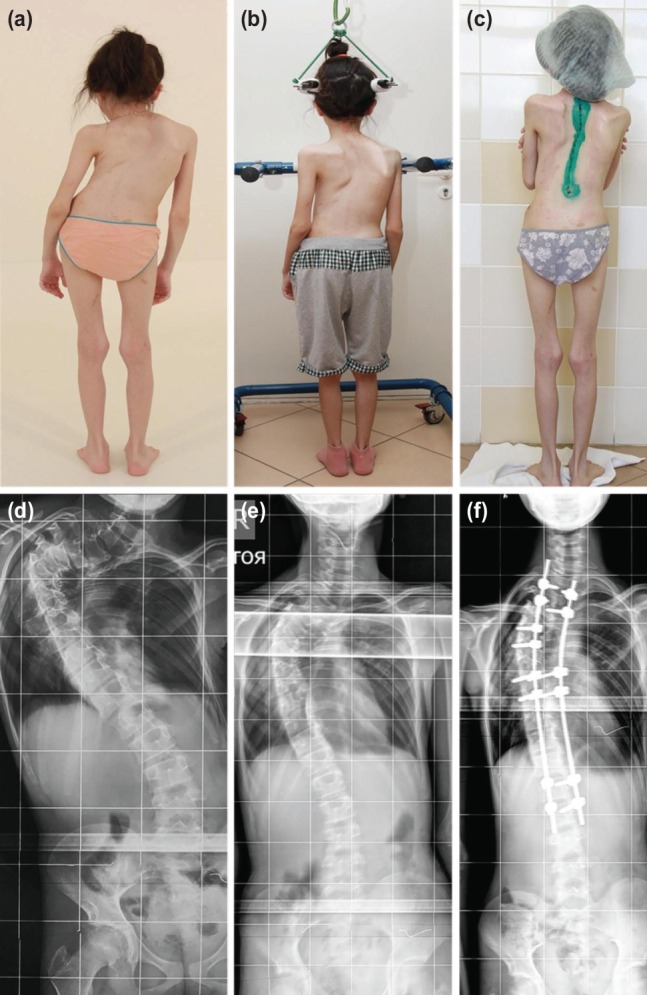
13-year old with Neurofibromatosis (Case 3). Images (a) pre-operative, (b) on traction and (c) post-operative. Radiographs (d) Pre-operative, (e) on traction and (f) post-operative.
